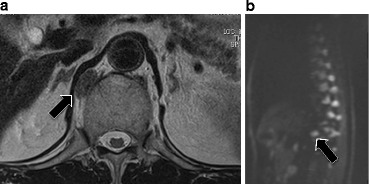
Paravertebral lymph node (a, *arrow*) is detected by MRI in T2. Paravertebral lymph node is high intensity in DWI (b, *arrow*)
